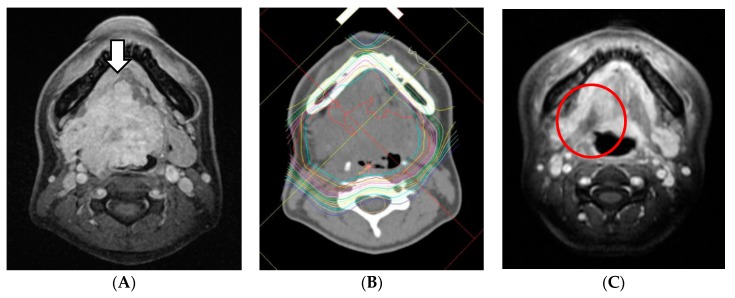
MRI of a patient, cT4aN0M1 (lung), before and after treatment. The tumor showed a CR but necrotic ulcer occurred. It was healed with conservative treatment. (A) Before treatment. White arrow: tumor. (B) The dose distribution of PBT. (C) After 2 months. Red circle: necrotic ulcer.
